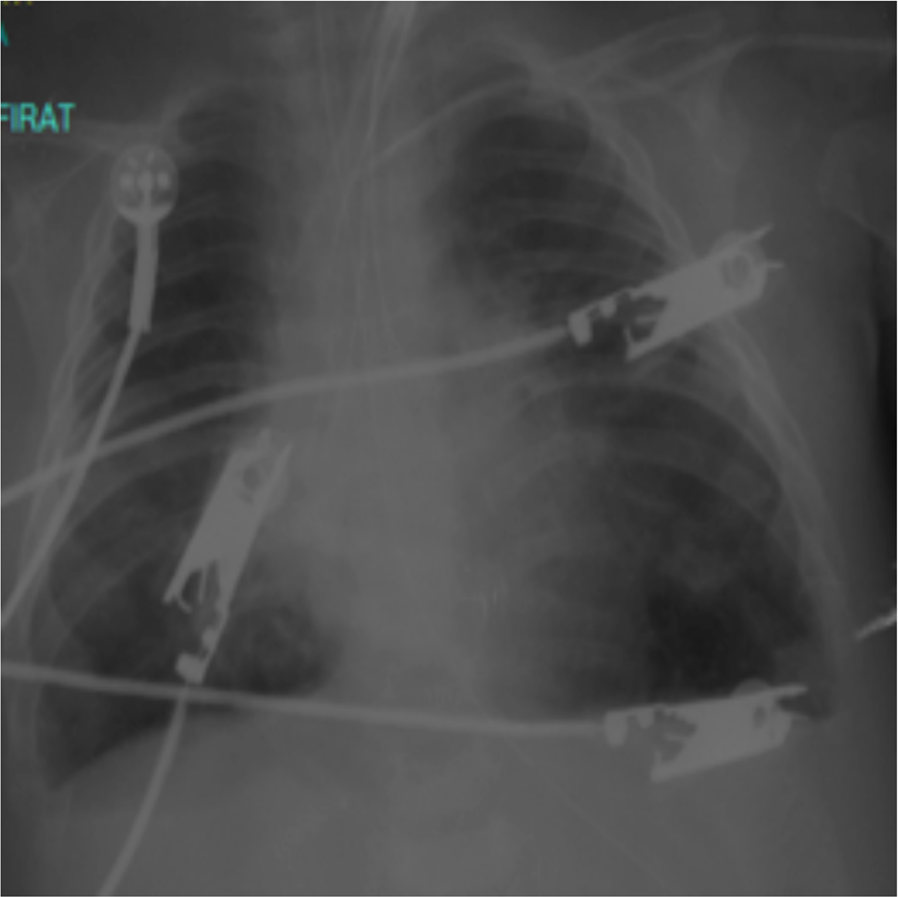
Chest radiographic image revealing that the atelectasis of the left lung and hyperinflation of the right lung were ameliorated after 12 hours of differential lung ventilation
